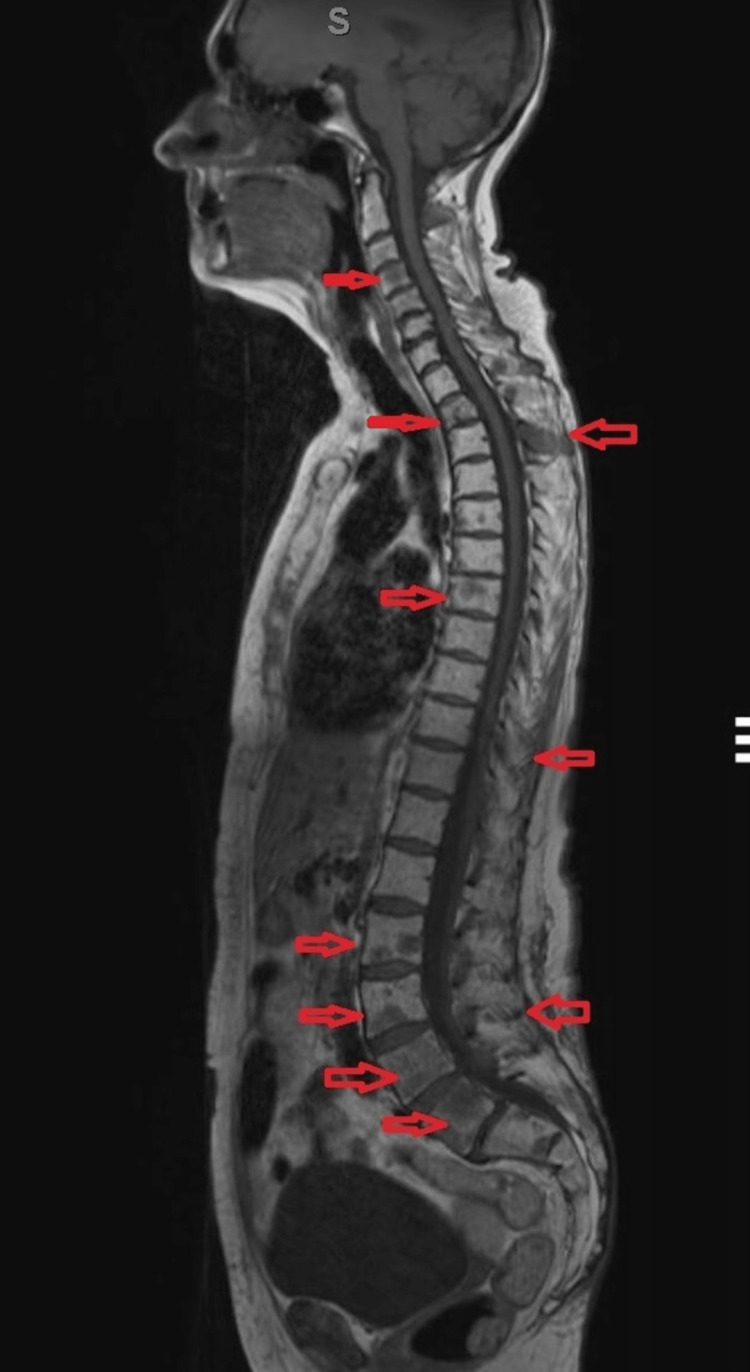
MRI lumbar-sacral spine T1-weighted image showing panspinal multiple myeloma lesions
